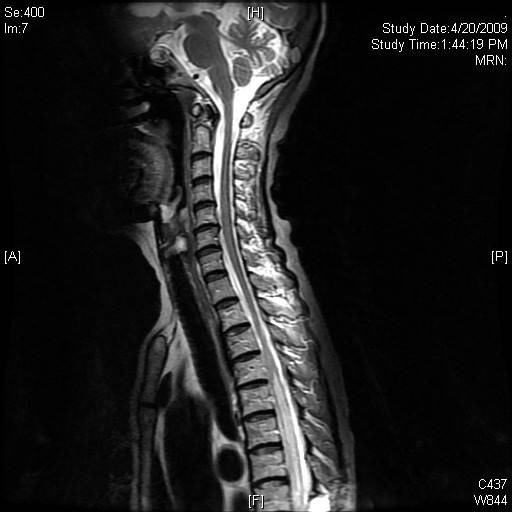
Selected sagittal MR image of the cervical and upper thoracic spine after treatment with methyprednisolone and resumption of chemotherapy, showed near resolution of the abnormal cord signal.
